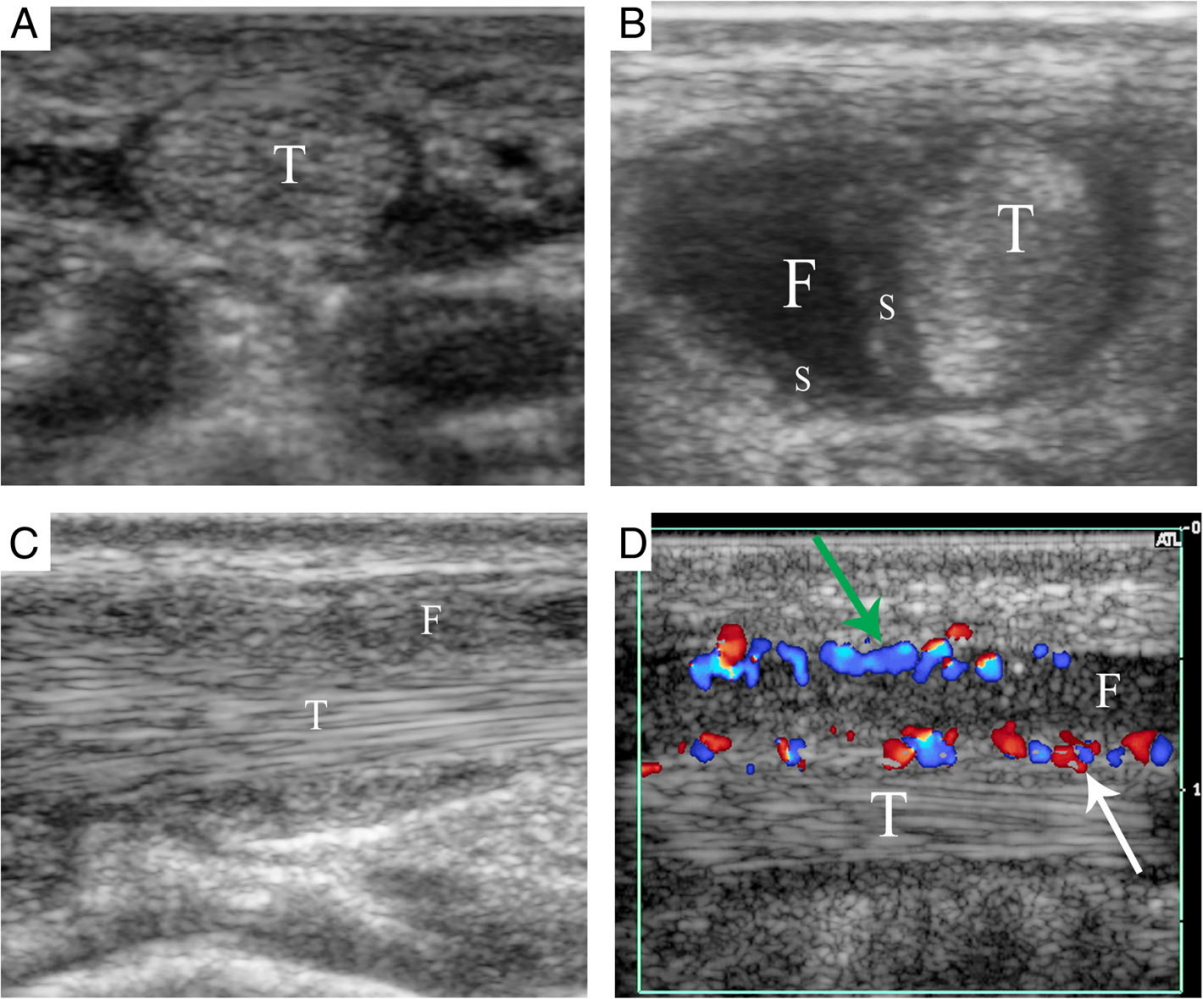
Sonographic findings of tenosynovitis in JIA. a 13 years 5 months old female referred for injection of the 4th flexor tendon sheath of the right hand. Ultrasound showed no fluid or signs of inflammation around the tendon in the transverse view. b 9 years 11 months old female referred for injection of the tibialis posterior tendon sheath. Ultrasound showed accumulation of peritendinous fluid and thickening of the synovial sheath in the transverse view. c 8 years 1 month old female referred for injection of the tibialis posterior tendon sheath. Ultrasound showed accumulation of echogenic peritendinous fluid in the longitudinal view. d 15 years 8 months old male referred for injection of the tibialis posterior tendon sheath. Ultrasound showed peritendinous fluid, synovial thickening with increased color-Doppler signal on the synovium covering the tendon (*white arrow*) and lining the cavity (*green arrow*). T = Tendon, S = Synovial sheath thickening, F = Peritendinous fluid
